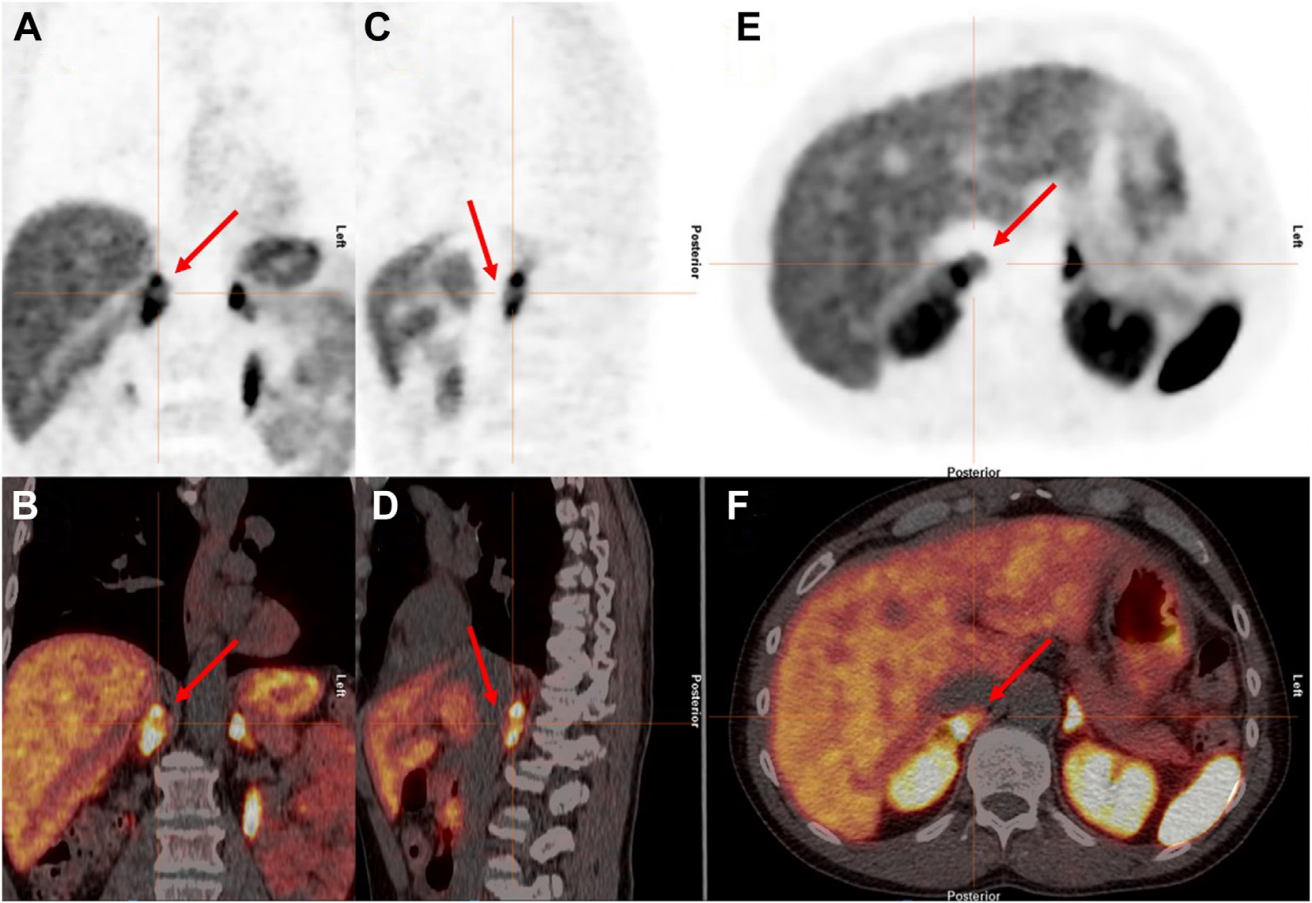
^68^Ga-DOTATATE PET/CT images. *A* and *B,* Coronal view PET. *C* and *D,* Sagittal view PET. *E* and *F*, Transverse view PET. The pheochromocytoma showed maximum standardized uptake value (SUVmax) of 7.73, which was lower than the surrounding normal adrenal tissue SUVmax of 17.23. There is a photopenic area (red arrow) within the right adrenal gland that reflects the location of the pheochromocytoma. *CT* = computed tomography; ^*68*^*Ga-DOTATATE* = Gallium-68 DOTATATE; *PET* = positron emission tomography.
